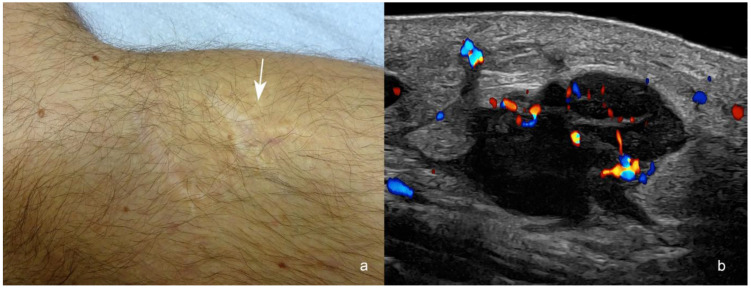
(a,b). Melanoma in-transit metastasis. (a). Clinical image shows the scar of the melanoma surgery and the location of a palpable pseudonodular structure in the posterior part of the medial aspect of the knee (arrow). The melanoma was originally located in the anterior part of the scar. (b). Color Doppler ultrasound at 24 MHz (longitudinal view) presents a hypoechoic hypodermal mass with spiculations and lobulations at 3 cm posteriorly from the primary tumor location (arrow in (a)). Notice the increased echogenicity of the adjacent hypodermis and the prominent vascularity within the mass. There is also a “tail sign” in the upper and lower borders of the nodule.
